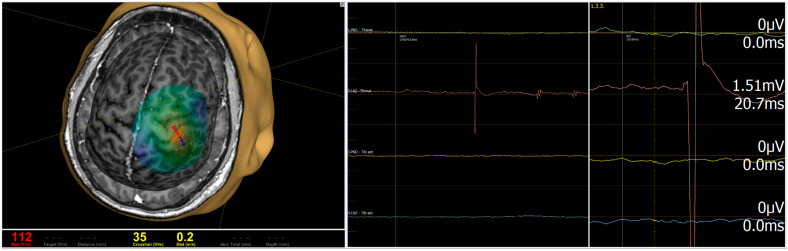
Single-pulse mapping of motor areas. Example of navigated transcranial magnetic stimulation (nTMS) motor mapping using the Nexstim NBS System 5, software version 5.2.4 (Nexstim Plc, Helsinki, Finland). The stimulation site (red vector) elicited a motor-evoked potential (MEP) in the contralateral upper limb. The color-coded activation map shows the cortical representation of the hand area overlaid on an individual MRI. The red vector indicates the stimulation site and coil orientation/trajectory. The colored traces on the right represent EMG/MEP recordings from monitored muscles.
